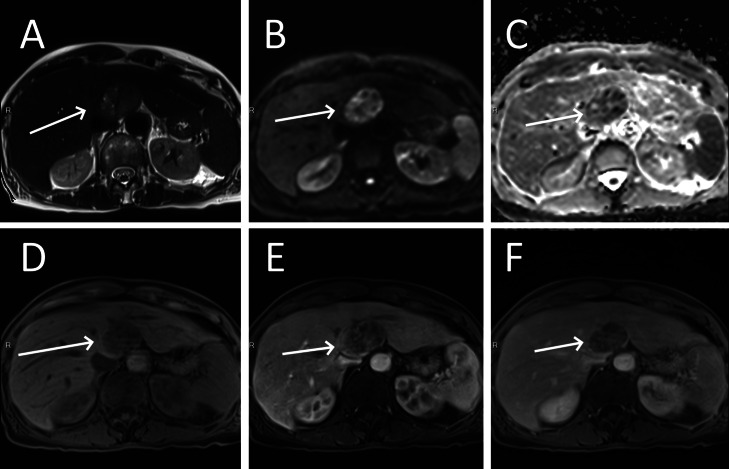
MRI of a hepatic metastasis (arrows) that is heterogeneously hyperintense in T2-weighted imaging (A), restricts diffusion on high b-value(B), is hypointense in ADC imaging (C), is hypointense to the liver in T1-weighted imaging (D) and enhances heterogeneously in arterial (E) and venous phases (F).
